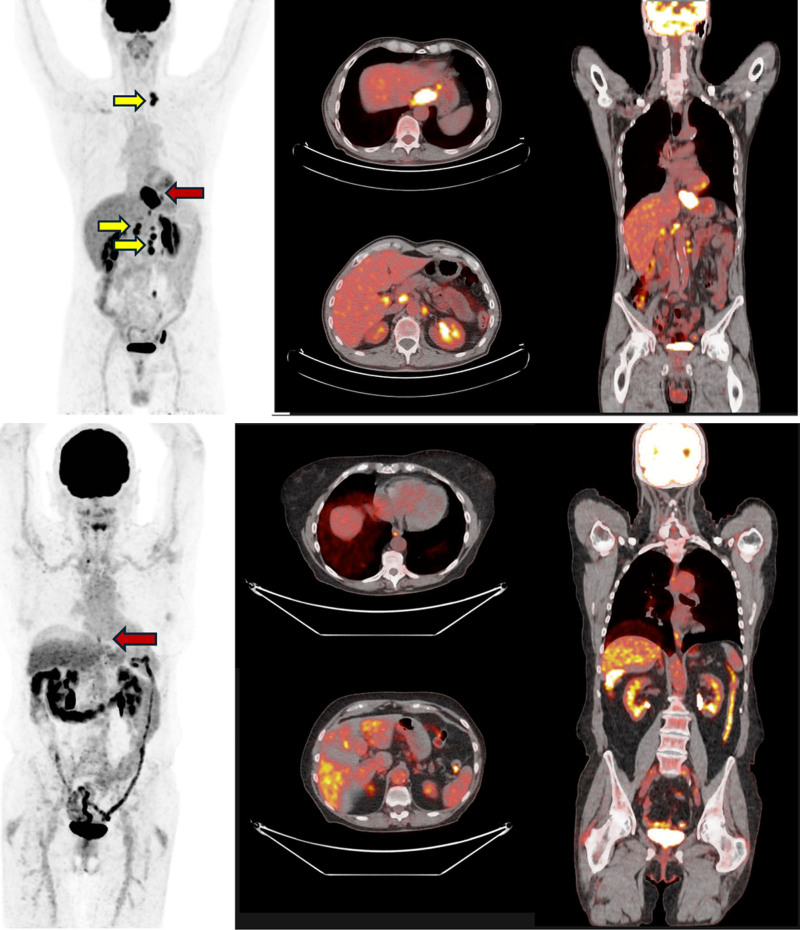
Baseline 18F-FDG PET/CT imaging for case 1 (upper) and case 2 (lower).
